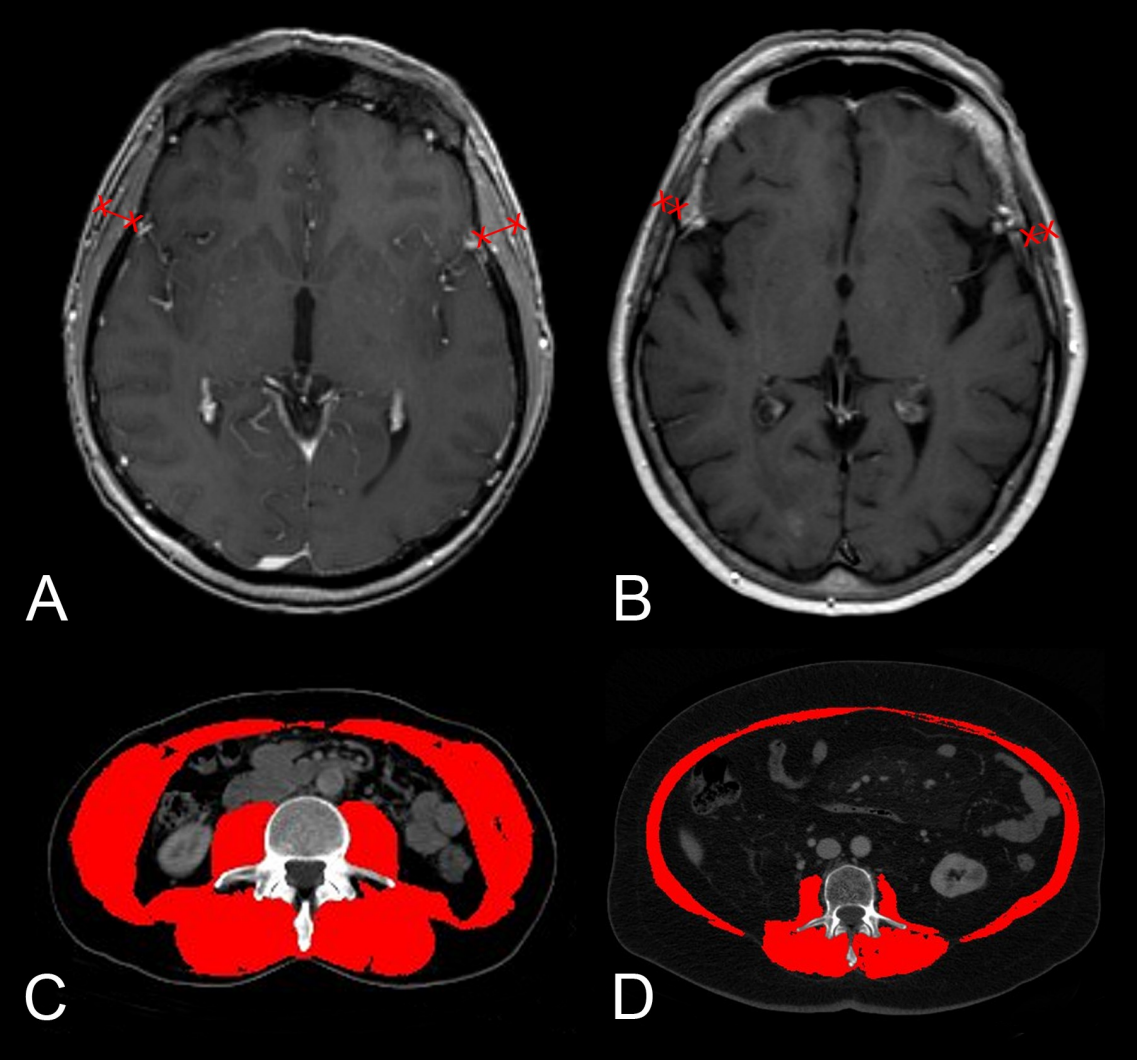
Examples of CSA measurements on CT images (A, C) and TMT measurements on brain MR images (B, D) in two melanoma patients with brain metastases. A, B, a male patient with an estimated normal skeletal muscle mass (mean CSA = 191.61 mm^2^; mean TMT = 7.3 mm) and C,D, a female patient with considerable estimated skeletal muscle mass loss (mean CSA = 106.07 mm^2^; mean TMT = 4.5 mm).
